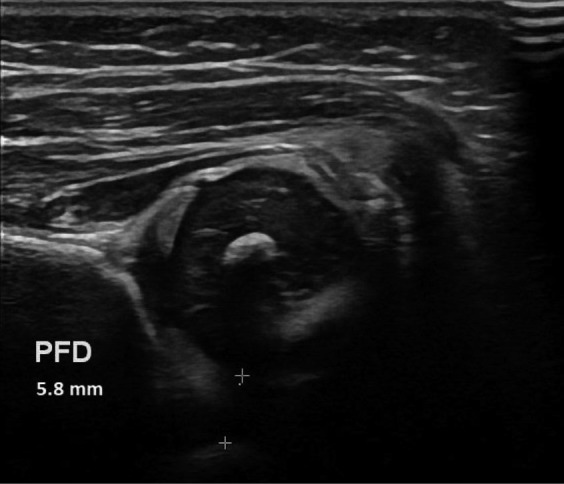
Ultrasound image of abnormal hip, girl aged 4 weeks, all anatomical landmarks present. PFD estimated at 5.8 mm as indicated by the 2 + symbols.
